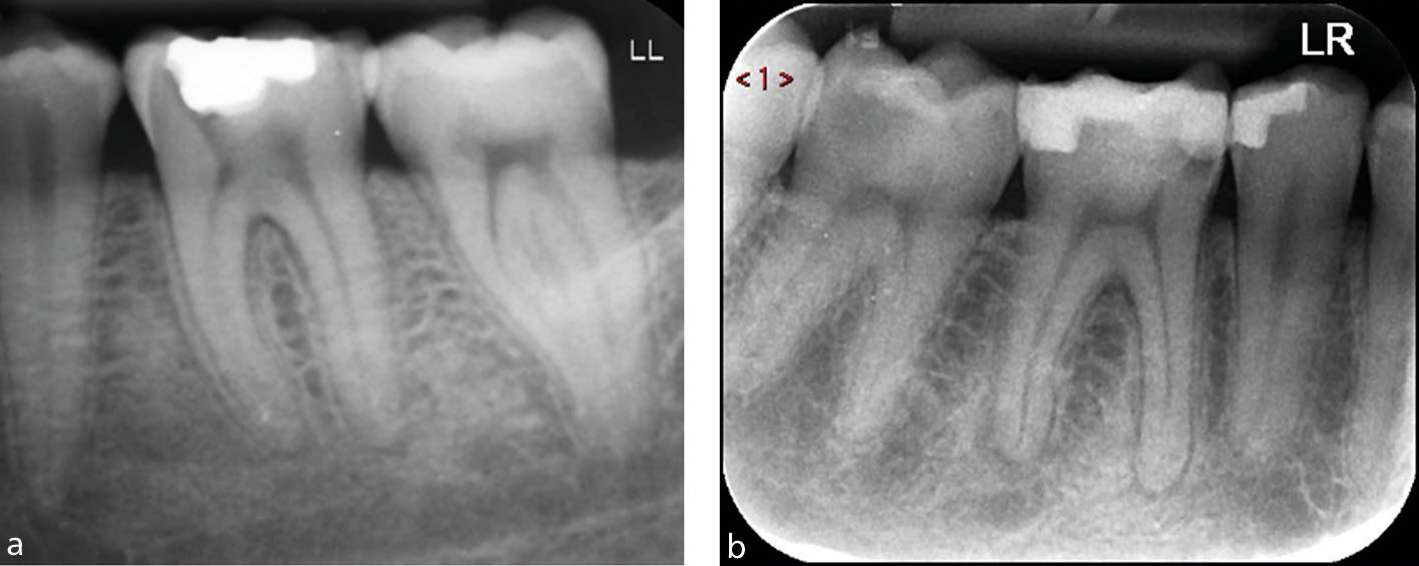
a, b) Periapical radiographs showing the 36 and 46, in both cases requiring endodontic treatment, with large, visible pulp chambers. This reduces the complexity of access cavity preparation, as the pulp chamber and canal orifices will be more easily located
